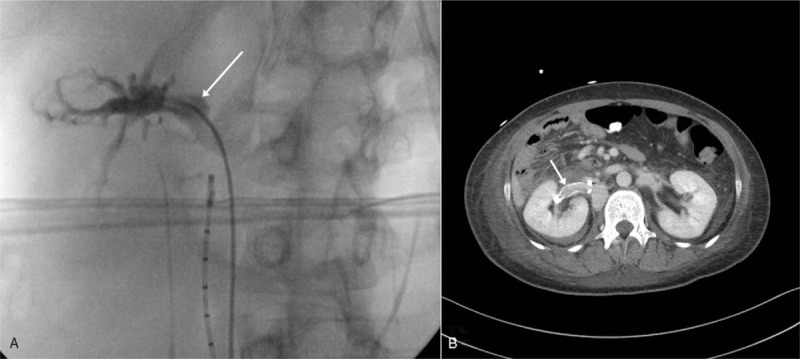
(A) C-arm fluoroscopic venography revealed the presence of a venous pseudoaneurysm as evidenced by filling of the contrast medium (white arrow). (B) Follow-up abdominal computed tomography scan performed 1 wk after stent graft insertion showed the stent in the right renal vein (white arrow) and a smaller perinephric hematoma.
